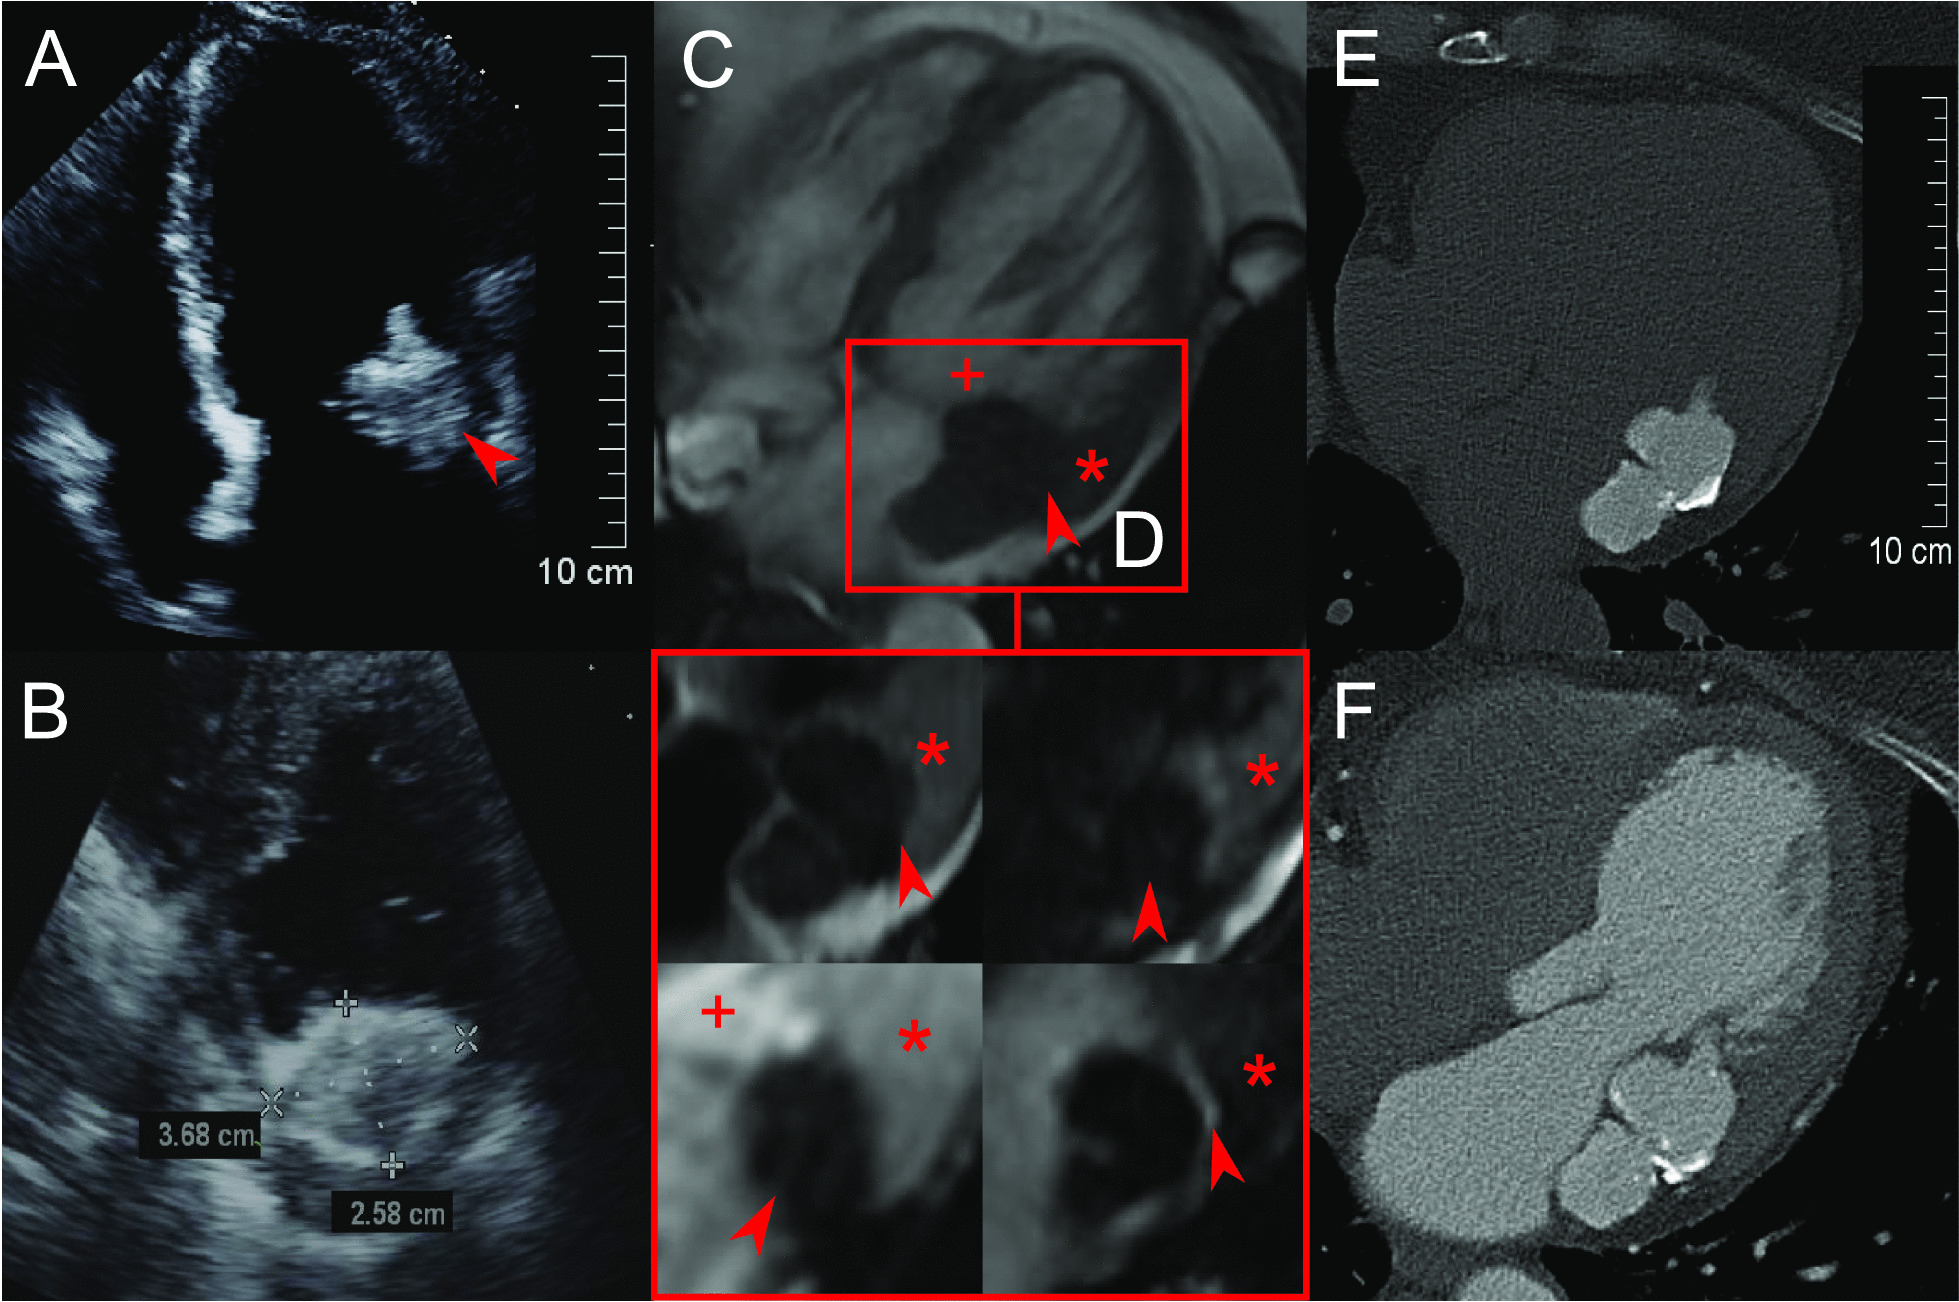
Exemplary characteristics of CMAC (case 6). Echo: apical long axis view A with the hyper-echoic mass (arrow) at the lateral mitral valve annulus with acoustic shadowing; short-axis view B with a blurred demarcation of the hyper-echoic capsule and hypo-echoic core. MRI: cardiac 4-chamber view in steady state free precision sequence C with the iso-intense mass (arrow) to the adjacent myocardium (star), but with a clear hypo-intense demarcation from intracavitary blood (plus sign); T1-weighted sequence with the hypo-intense mass (arrow) and less intense core in T2-weighted sequence (arrow) compared to the myocardium (star) (D; top left and top right); first pass perfusion with low signals of vascularisation compared to myocardium (star) and intraventricular blood (plus sign) (D; bottom left); late gadolinium enhancement with very low signal in the core, but peripheral bright border (arrow) (D; bottom right); CT: precontrast hyperattenuation of the CMAC mass with peripheral calcifications (E) and no accumulation of contrast agent (D). *CMAC* caseous mitral annular calcification, *Echo* transthoracic echocardiography, *MRI* cardiac magnetic resonance imaging, *CT* cardiac computed tomography
